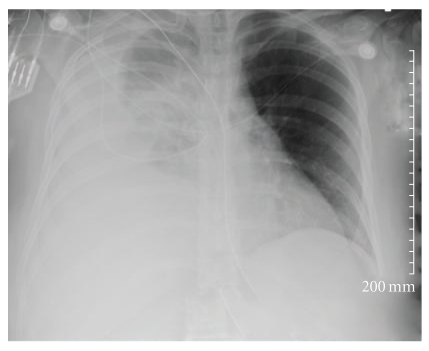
Chest X-ray showing right hemithorax opacification.
